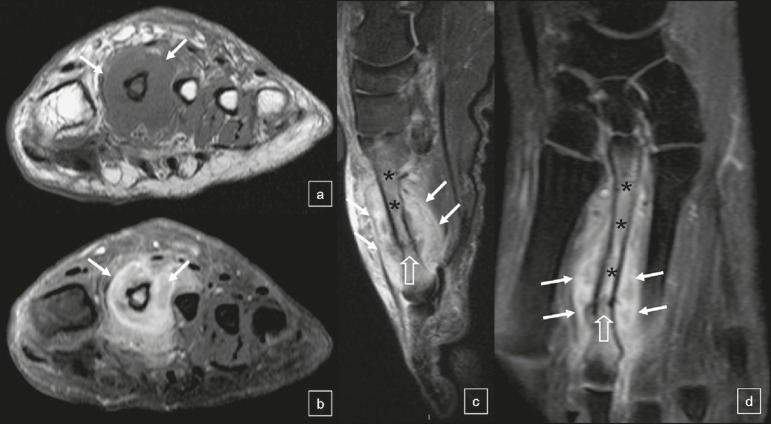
MRI of the left foot. Gadolinium contrast-enhanced axial T1-weighted MRI
